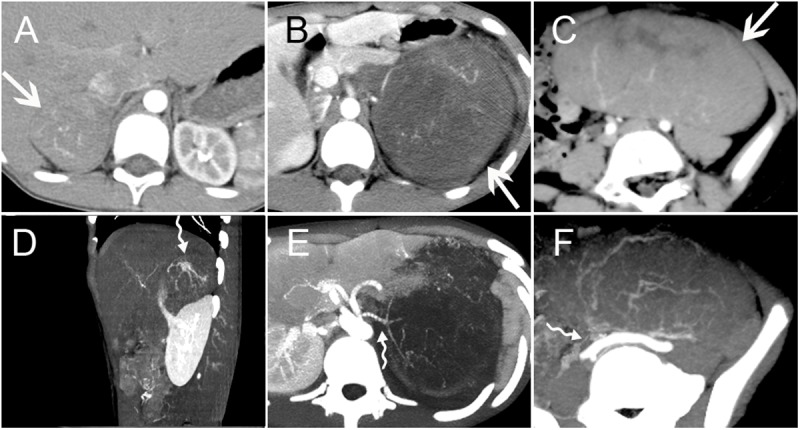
Tumor Blood Supply. Three PNET cases with masses arising in the right adrenal gland region (A/D) (Case no. 2), left kidney (B/E) (Case no. 17) and mesentery (C/F) (Case no. 14) (arrows). Contrast-enhanced arterial phase CT images showed circuitous lines of small blood vessels within the masses. Tiny feeding arteries could be observed inside the mass, showing a crab-like appearance (curved tail arrow) (D-E).
